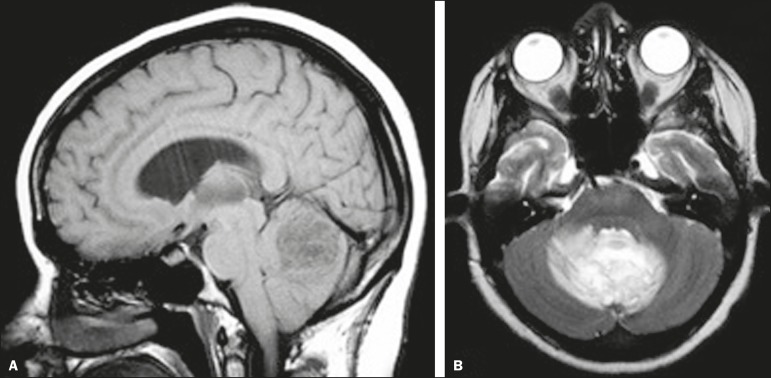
Case 18.* Cryptococcoma. Sagittal T1-weighted image showing a
